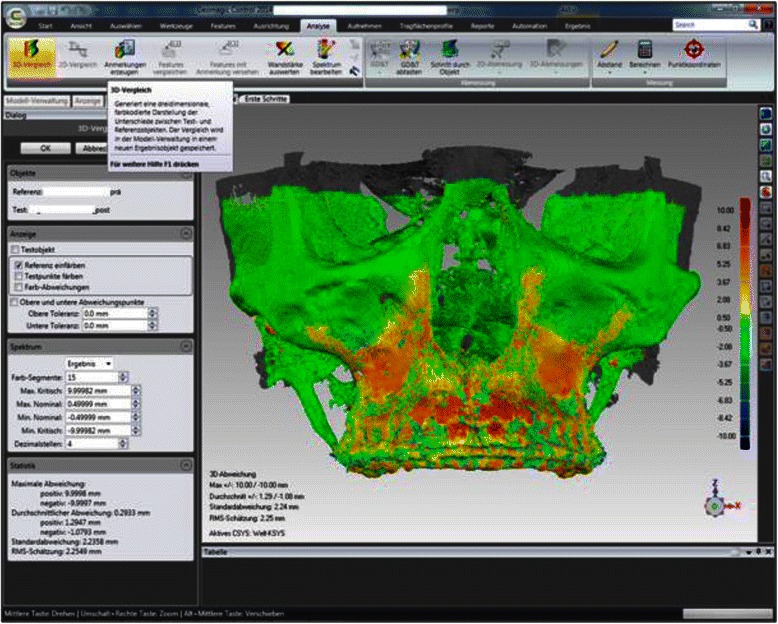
The user interface in Geomagic Control after completion of the superimposition of the cone-beam computed tomograms from T0 and T1. The colors diverge from green to show the skeletal changes
